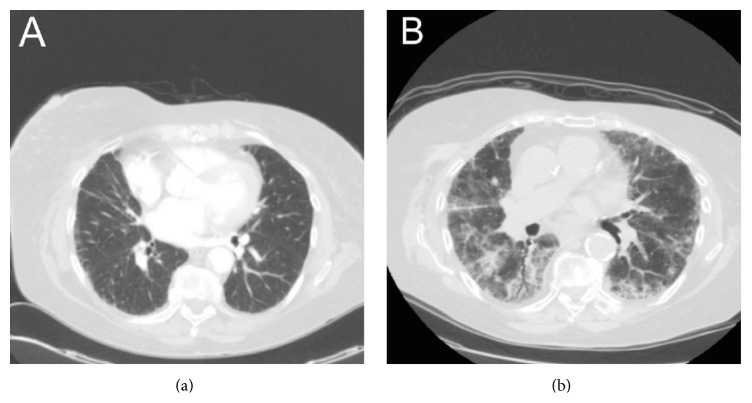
Chest CT scan at baseline (a) and after second course of chemotherapy administration, with the replacement of lung tissue by connective tissue (b).
